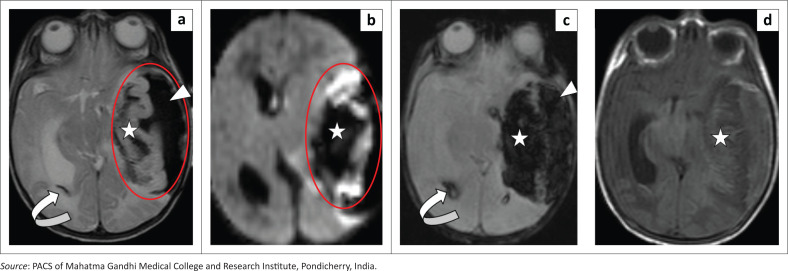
Axial MRI sequences. T2-weighted (a), diffusion-weighted imaging (DWI) (b), gradient recalled echo (GRE) (c) and T1 weighted (d) reveal left temporal T2 hypointense subpial haemorrhage with blooming on GRE. Left temporal lobe intraparenchymal extension (star), intraventricular extension and hydrocephalus noted (curved arrow). The T2-weighted and DWI sequences demonstrate the classic ‘yin-yang sign’ of subpial hypointensity and underlying cortical hyperintensity (circled in red).
